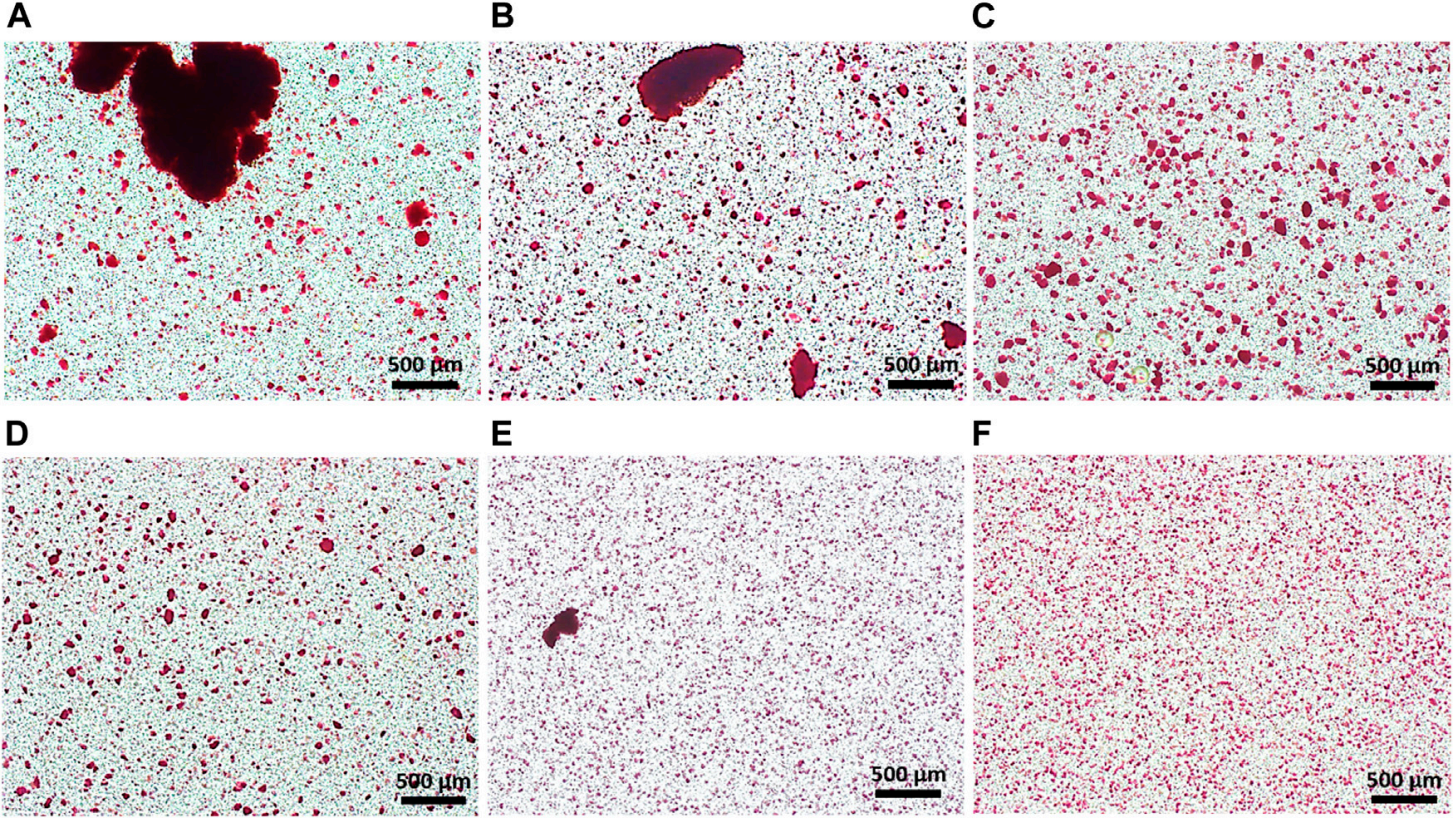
Homogeneity of HAp powder in alginate solution; stirring for (A) 6 min and (B) 21 min; planetary centrifugal mixing for (C) 6 min and (D) 21 min; 3D ultrasonic mixing for (E) 15 min (F) planetary centrifugal mixing for 6 min and 3D ultrasonic mixing for 15 min.
